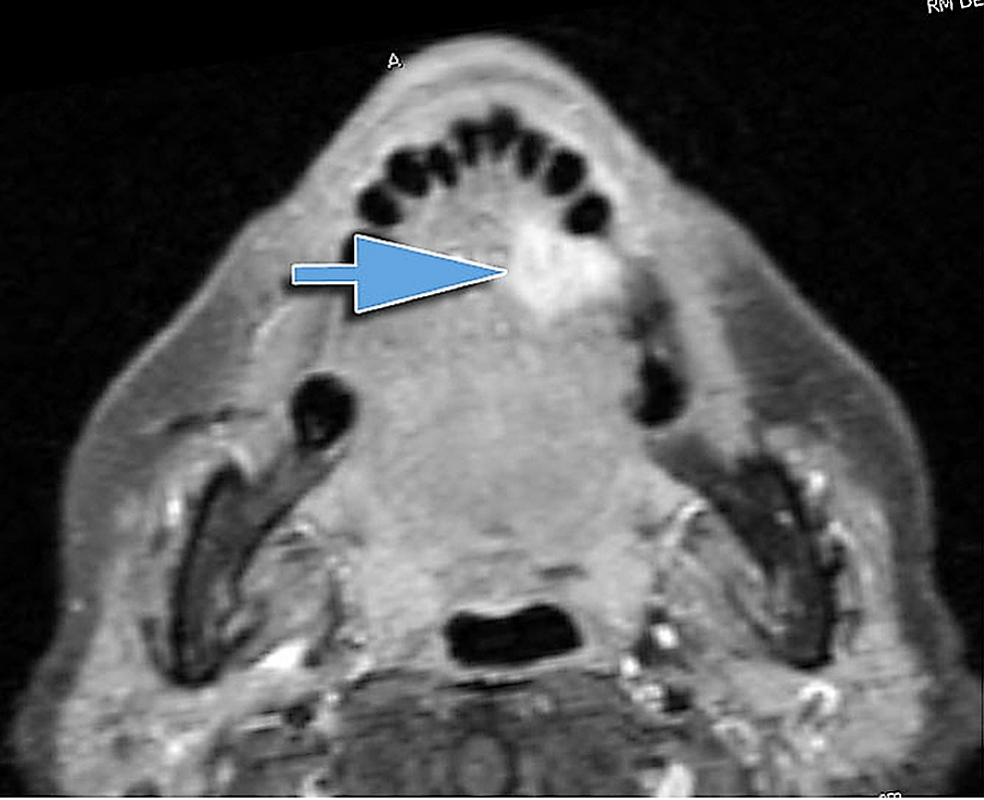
Squamous cell carcinoma of the left tongue. The MRI shows an extension in depth greater than what is visible. The neoplasm approaches very close to the midline (arrow) but does not reach it. MRI: magnetic resonance imaging
